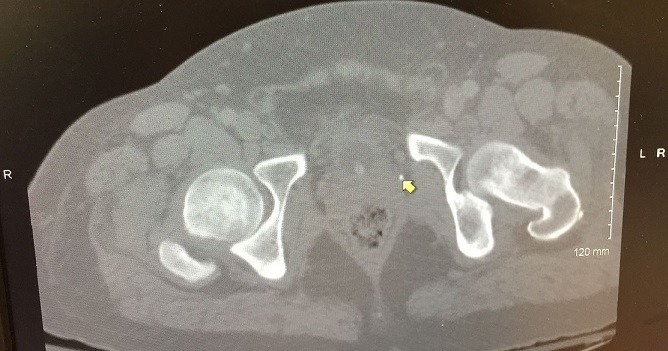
CT-scan abdomen and pelvis (axial section), showing rim enhancing fluid collections in the left obturator foramen approximately 2.5cm in diameter consistent with an abscess (arrow); there is some abnormality in the mesorectal fat along with some stranding extending up from the lower left pelvis along the iliac chain anterior to the psoas
